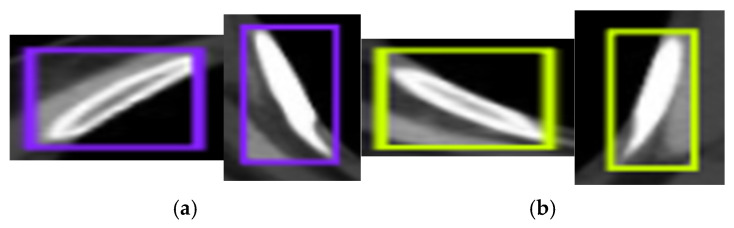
Examples of labeled regions on CT images: (a) labeled representation of fractured regions and (b) labeled representation of non-fractured regions.
